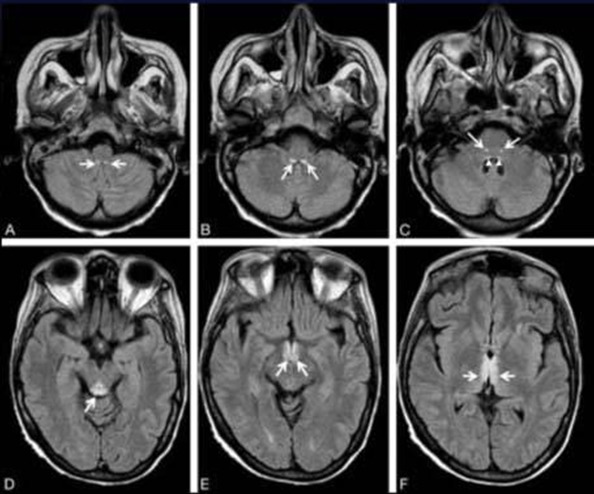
MRI of the head showing a diffuse hyper intensity of leptomeningeal spaces
